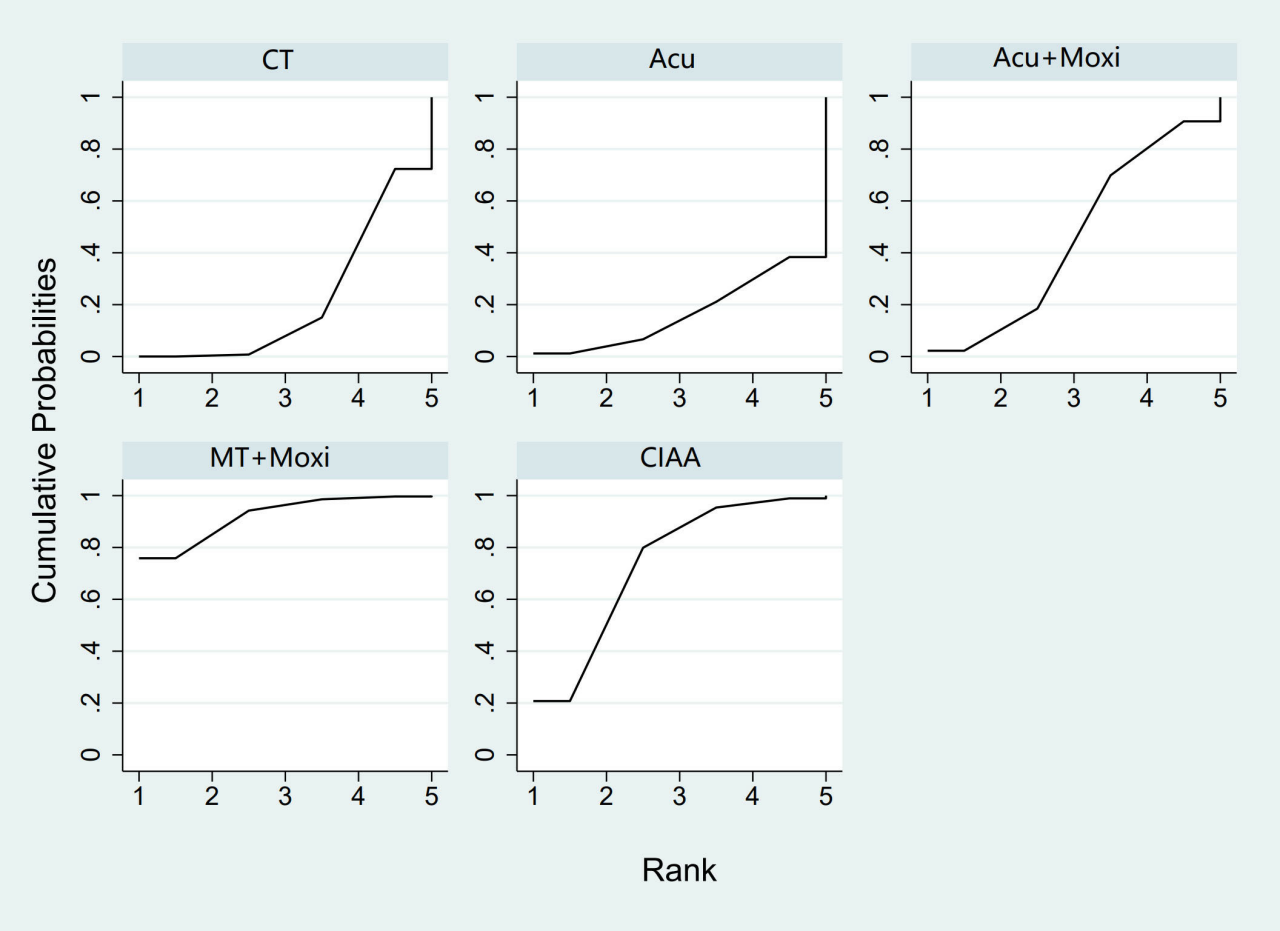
Efficacy ranking and cumulative probability graph of Kupperman scores treated by different types of intervention. CT, conventional therapy; Acu, acupuncture; Moxi, moxibustion; CIAA, catgut implantation at acupoint; MT, massage therapy.
